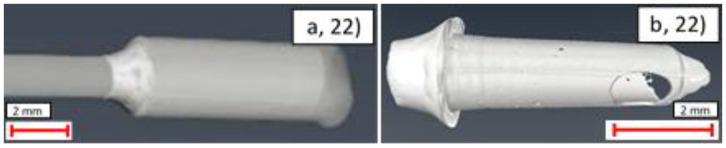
CT images of Ni + Cu-coated sample (22): (a) side view of pipe and gland exterior; (b) side view of right side interior solder.
